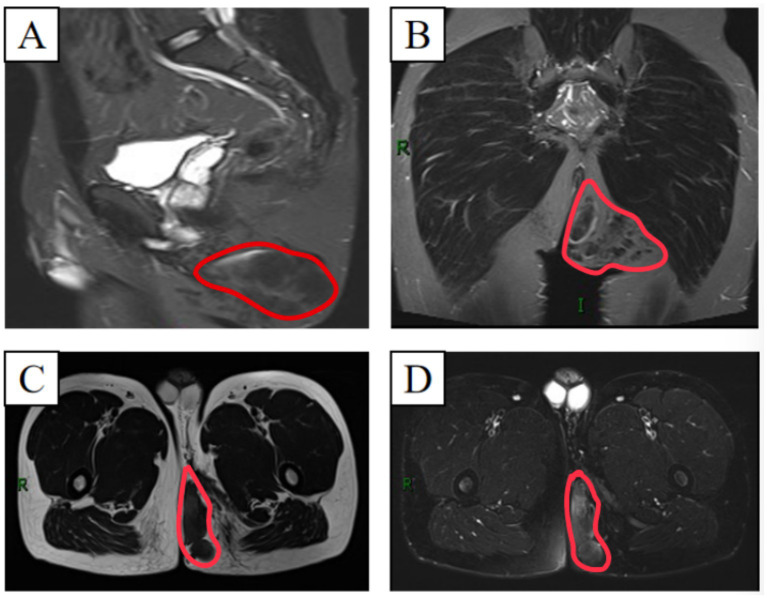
(A–D) Pelvic MRI indicated that the perianal tumor is located in the left gluteal cleft (red circle). Long T1 and slightly prolonged T2 signals, and the fat-suppressed sequence (T2WI-FS) showed a mildly high signal. Patchy long T2 signal shadows surround the lesion, and diffusion-weighted imaging showed a mildly high signal.
